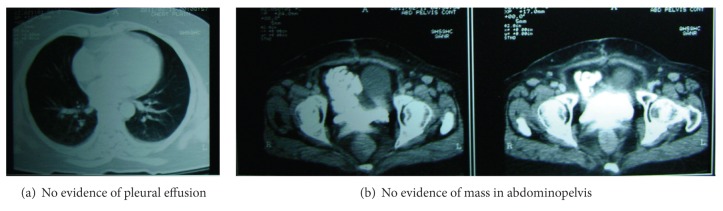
CT scan after 4 weeks following 6 cycles.
